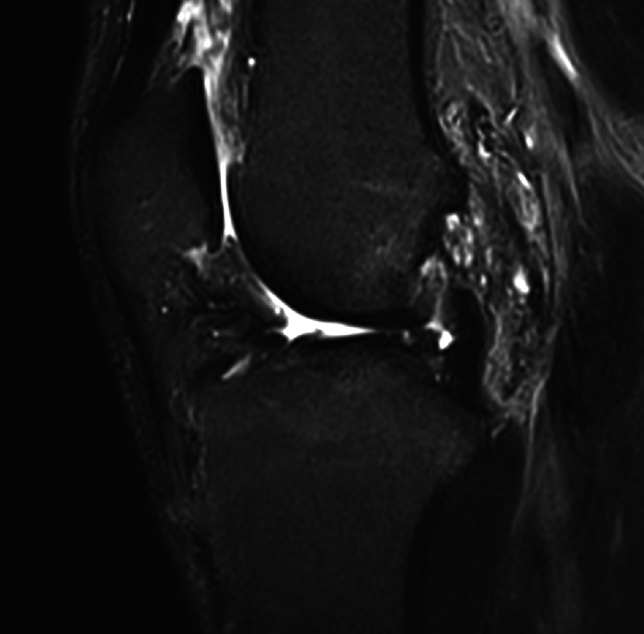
31-year-old male patient at follow-up MRI 7 months after QT autograft ACLR showing complete graft resorption and missing graft signal 6
